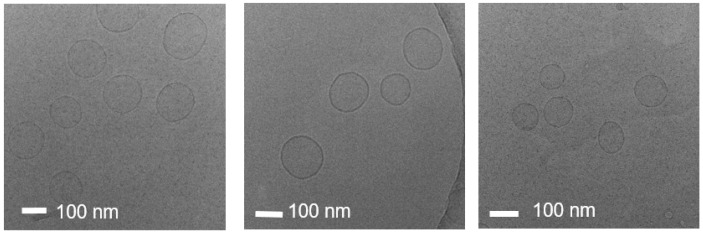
Cryo-EM images of liposomes prepared using focused ultrasound method.
